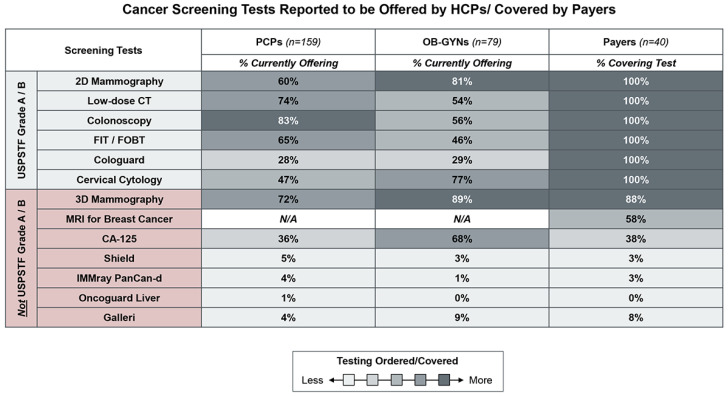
Cancer screening tests reported to be offered by HCPs/covered by payers. Survey respondents were asked which cancer screening tests they currently offer in their practice or are covered by their plans for HCPs and payers, respectively. The tests inquired about included those tests with USPSTF Grade A/B ratings, including 2D mammography, low-dose computed tomography (CT), colonoscopy, fecal immunochemical test/fecal occult blood test (FIT/FOBT), Cologuard, and cervical cytology and a sampling of tests without USPSTF Grade A/B ratings, including 3D mammography, MRI for breast cancer, cancer antigen-125 (CA-125), Shield (i.e., blood-based liquid biopsy for colorectal cancer), IMMray PanCan-D (i.e., blood-based liquid biopsy for pancreatic cancer), Oncoguard Liver (i.e., blood-based liquid biopsy for hepatocellular cancer) and Galleri (i.e., blood-based liquid biopsy for multi-cancer early detection). Note that all payers are required to cover cancer screening tests with USPSTF Grade A/B ratings < 10% of HCPs and payers reported to offer/cover available blood-based liquid biopsy tests today.
